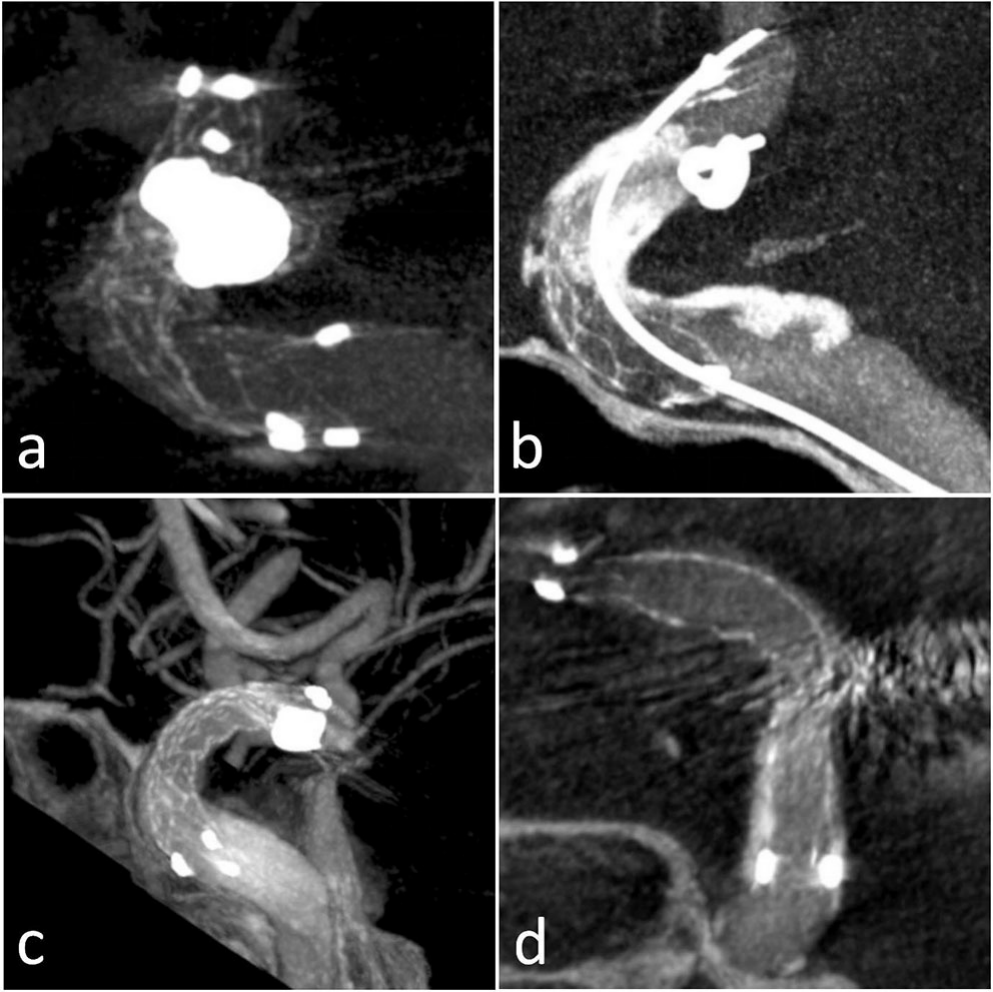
Four patients with ISA presentation in Xper CT: (a) Case 2 outer curve ISA; (b) Case 5 inner curve ISA; (c) Case 14 inner ISA; (d) Case 15 outer curve ISA.
